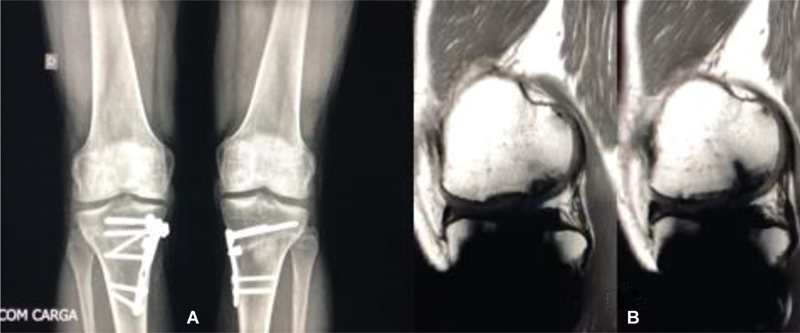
Postoperative radiograph after the bilateral osteochondral transplantation with consolidated osteotomies (operated 5 months apart) (
